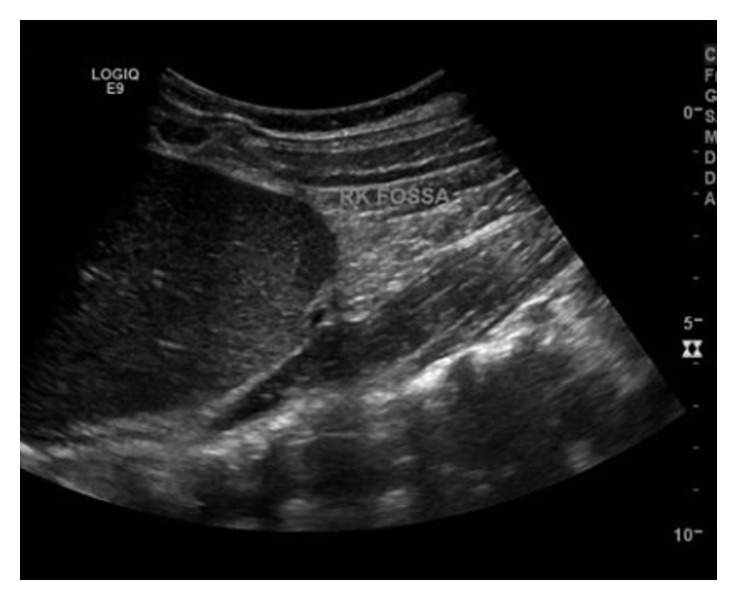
Long-axis/sagittal ultrasound image from the right upper quadrant showing no evidence of renal parenchyma.
